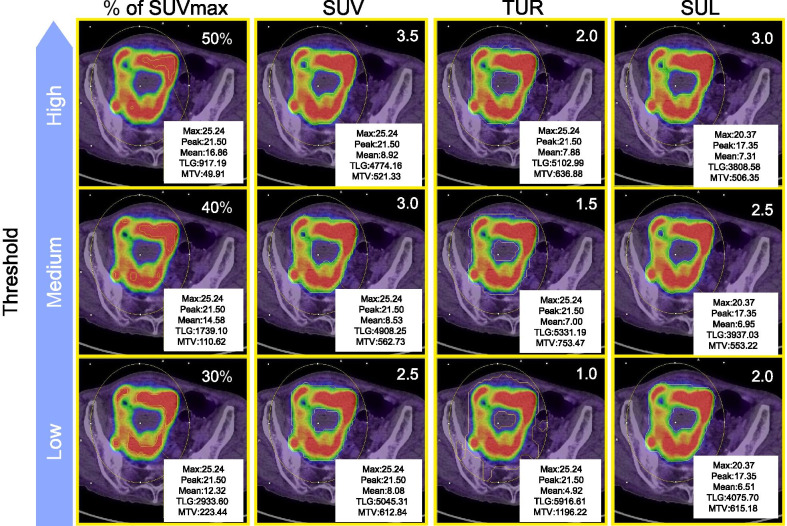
Thresholds in case of a higher SUVmax tumor. SUVmax, maximal standardized uptake value; SUV peak, peak standardized uptake value; SUV, mean standardized uptake value; MTV, metabolic tumor volume; TLG, total lesion glycolysis; TUR, tumor-to-liver uptake ratio; SUL, SUV normalized to lean body mass; VOI: volume of interest. VOIs in % of SUVmax were smaller than in other thresholds. For each threshold, the lower the value, the larger the VOI
